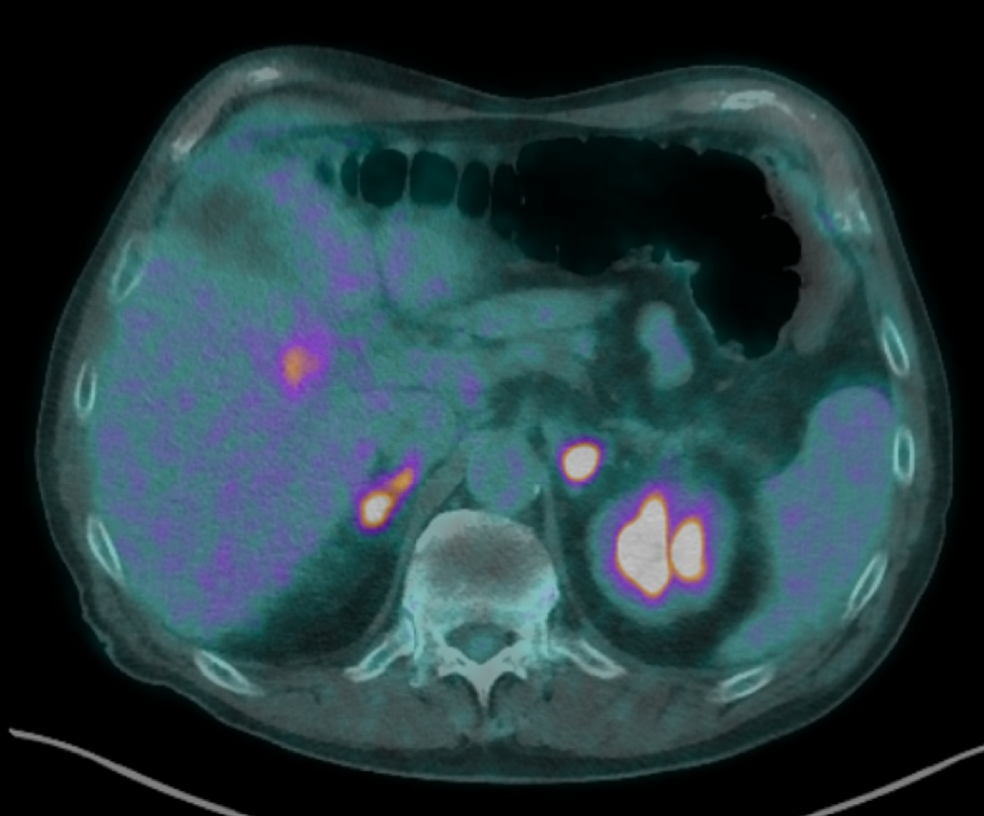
FDG-PET CT showing gallbladder fossa lesion FDG: fluorodeoxyglucose; PET: positron emission tomography
